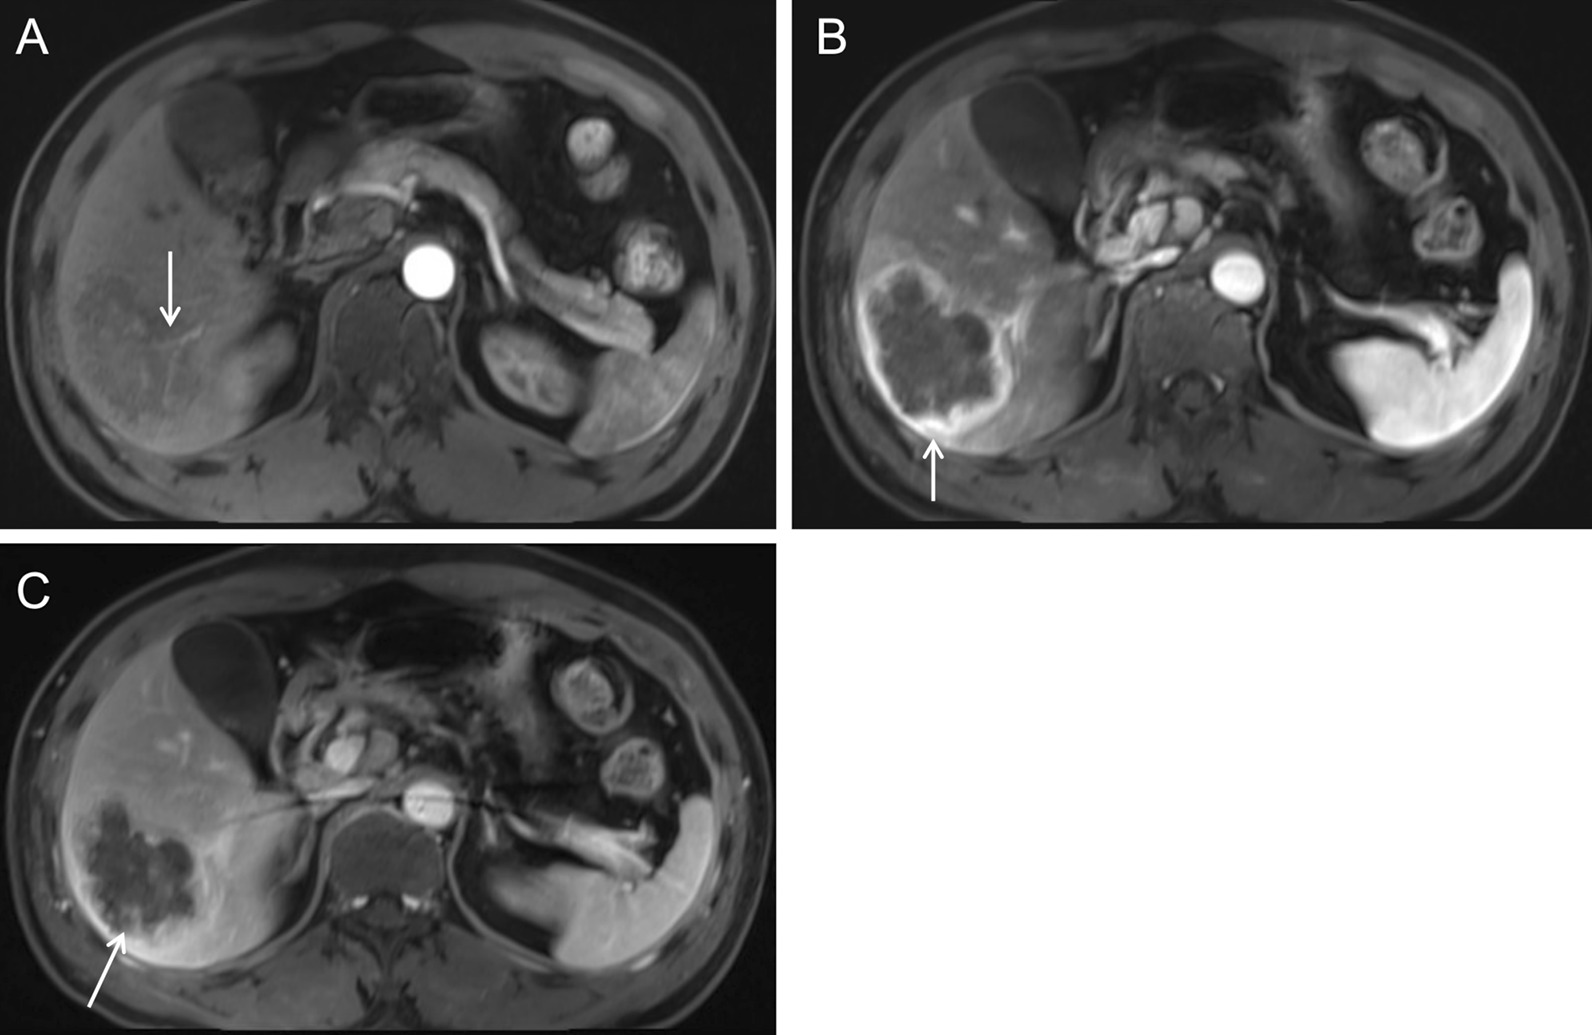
Enhanced MRI images of case 2
